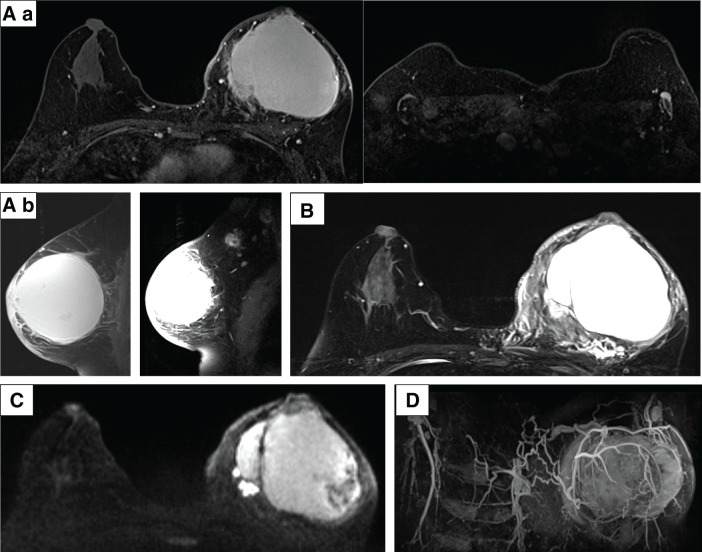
Contrast-enhanced MRI captured on cycle 2, day 22. (A) T1 (enhanced) rapid phase: (a) horizontal section (breast tumor, axillary lymph nodes) and (b) sagittal section (left: breast tumor, skin edema; right: breast tumor, axillary lymph nodes). (B) T2, (C) DWI, and (D) MIP. These findings suggest inflammatory changes in the breast tumor. DWI, diffusion-weighted imaging; MIP, maximum intensity projection
